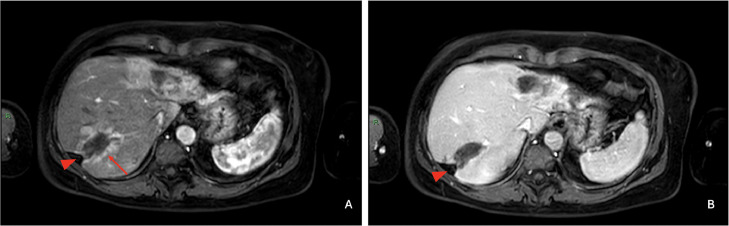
Magnetic resonance imaging with hepatospecific contrast agent. MRI showed the oblong alteration in the VII hepatic segment, causing retraction of the capsular profile (arrow head). This formation was characterized by irregular and progressive enhancement, predominantly peripheral, associated with hypervascular alteration in the arterial phase (arrow) (A) of the surrounding hepatic parenchyma, with isodensity in the later phases (B), attributed to THID (Transient Hepatic Intensity Differences).
